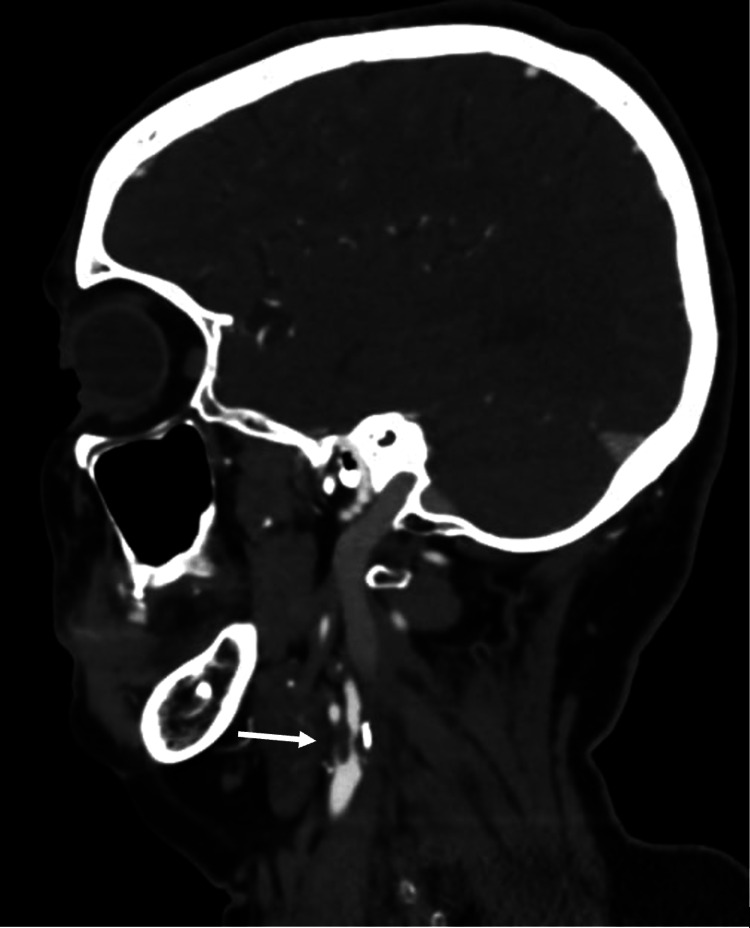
Computed tomography angiography showing severe luminal stenosis of around 90% involving the proximal segment of the right internal carotid artery associated with calcified plaque.
